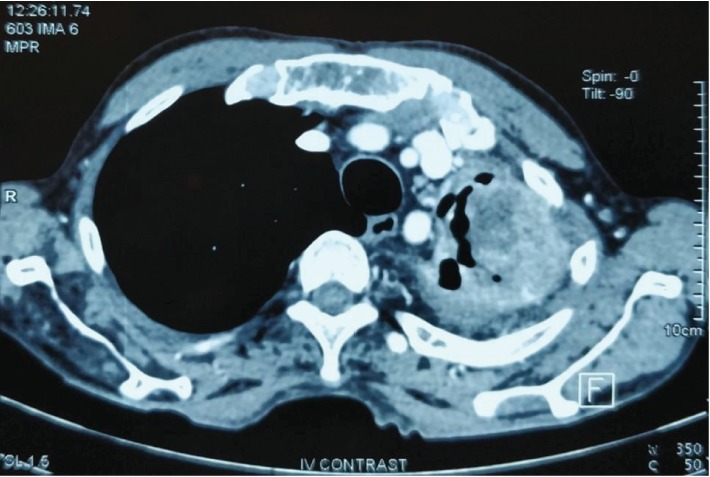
CT scan (mediastinal window) showing an enhancing lesion at the apicoposterior segment of the left upper lobe measuring 6.8 × 4.8 × 3.8 cm.
